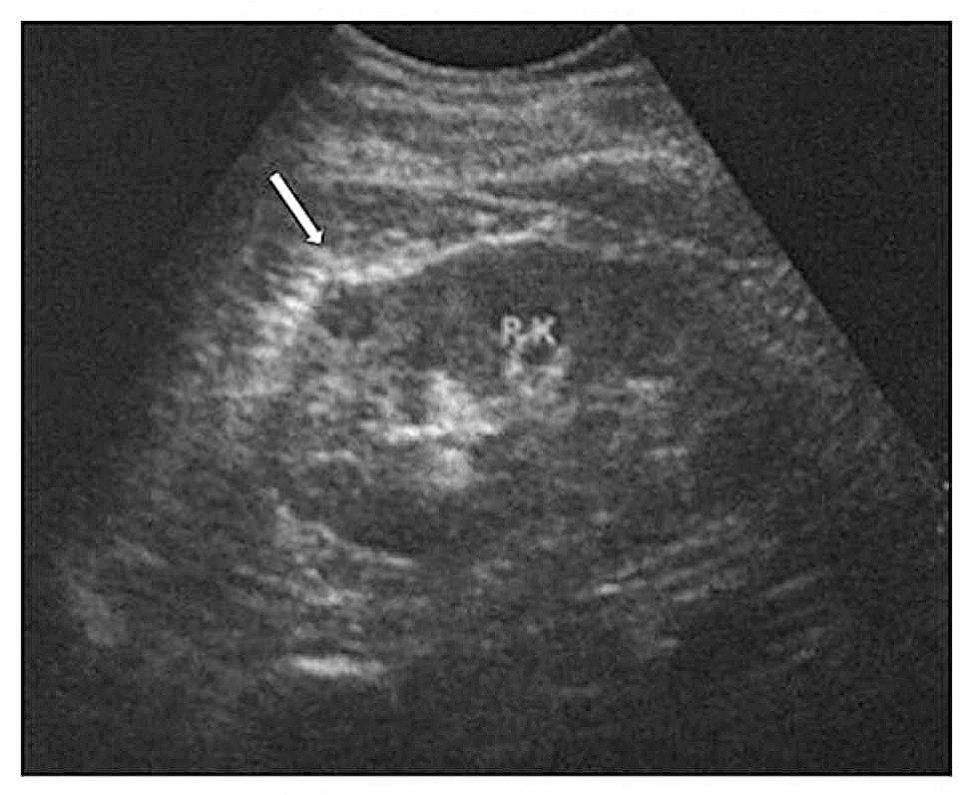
A JGCT lesion in the right kidney. Ultrasound showed extremely hypoechoic lesion (arrow), 1.2 × 1.0 × 0.8 cm,with regular morphology and clear boundary
